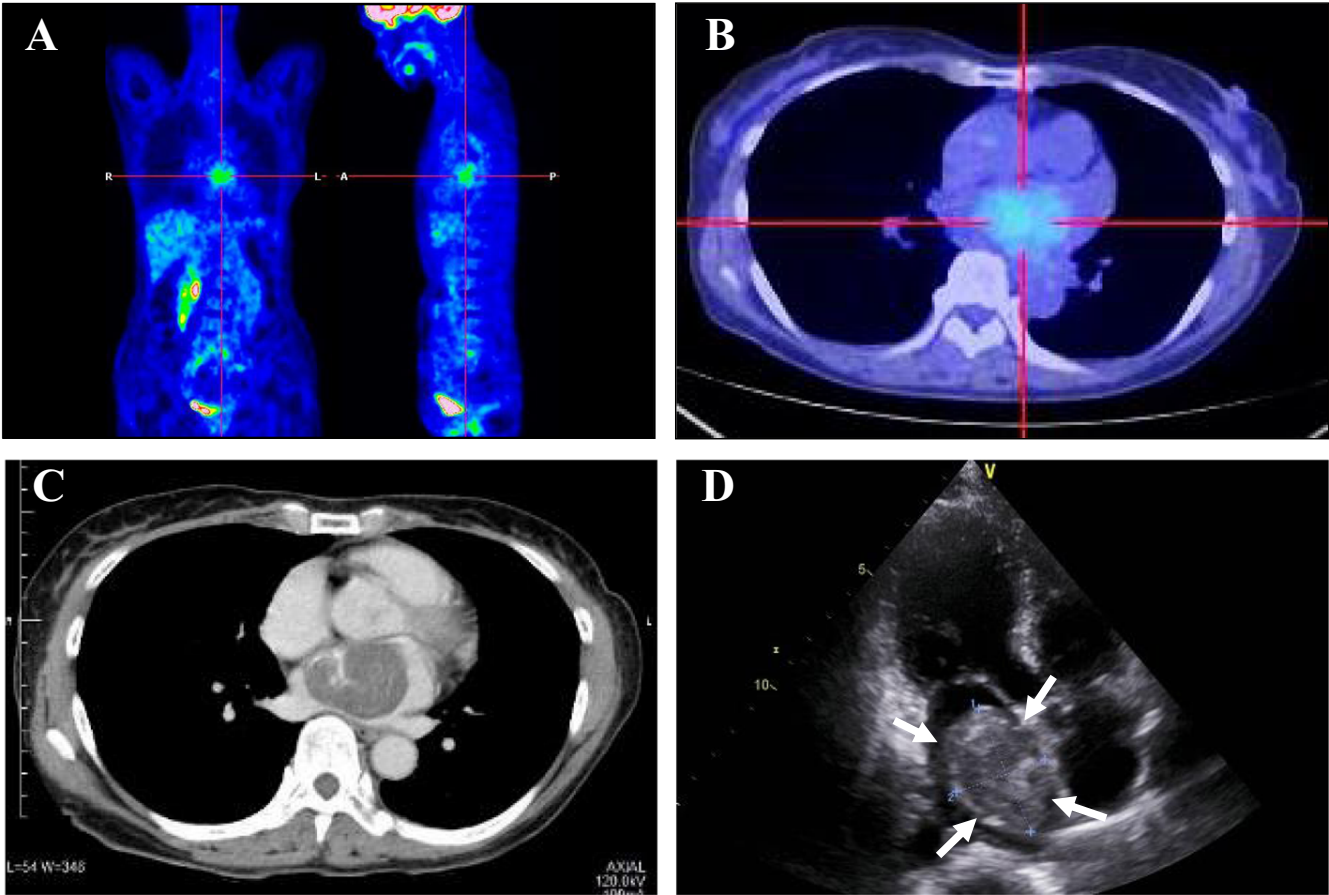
The findings of^18^ F-FDG PET/CT and echocardiography at surgery of the resected cardiac myxoma. (A–C) Coregistered ^18^ F-FDG PET/CT images in coronal (A) and axial (B) plane demonstrated a large and mildly to moderately hypermetabolic area in the left atrium (maximal SUV: 3.0), which corresponded to a hypodensisty mass lesion on chest CT (C), measuring 46 × 32 mm in diameter. (D) Subsequent transthoracic echocardiography revealed a pedunculated mass originating from the interatrial septum (arrows).
